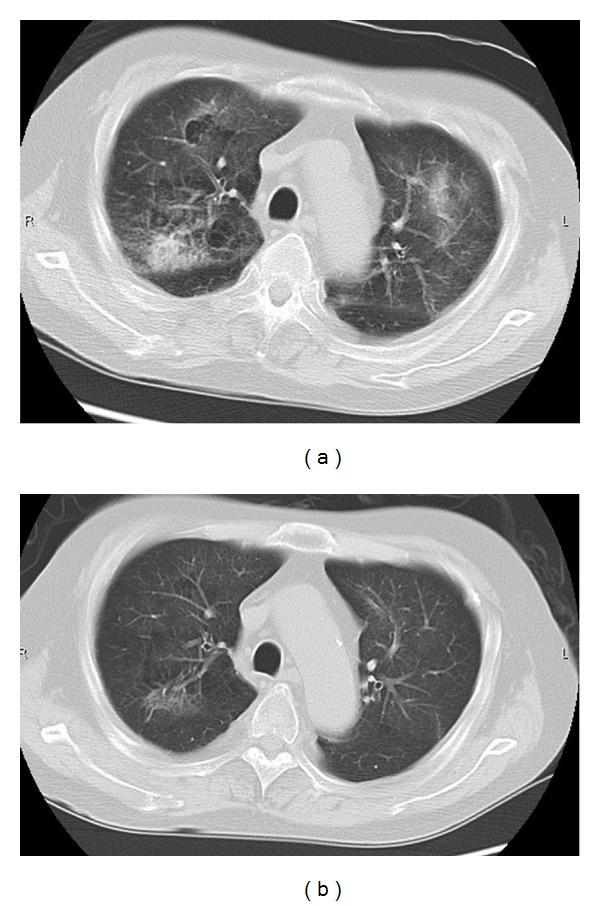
(a) Computed tomography scans on the day patient diagnosed as PCP showed infiltrates and reticular shadows. (b) The shadows disappeared after treatment with antifungal agents.
